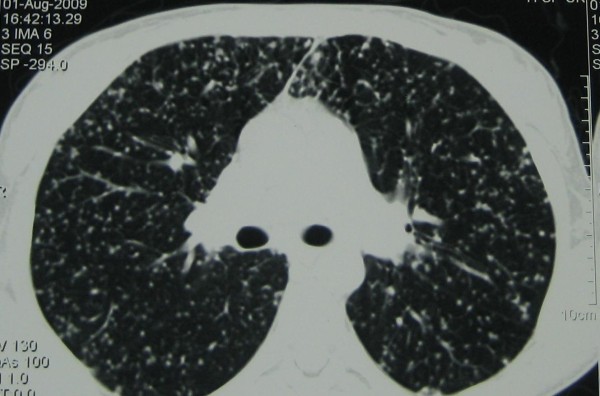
CT scan shows the typical radiological presentation of miliary tuberculosis.
